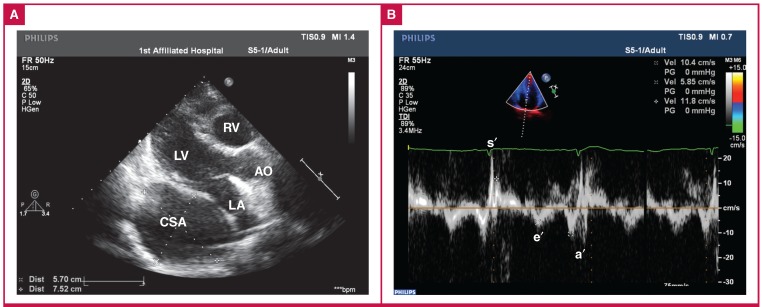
Transthoracic echocardiography on presentation showed: A. the appearance of the giant aneurysm of the coronary sinus in the parasternal long-axis view; B. tissue Doppler imaging velocities at the septal mitral annulus: early diastolic myocardial velocity (e′) was 5.85 cm/s and systolic myocardial velocity (s′) was 10.4 cm/s. RV, right ventricle; LA, left atrium; LV, left ventricle; CSA, coronary sinus aneurysm; AO, ascending artoa, a′, late diastolic myocardial velocity.
